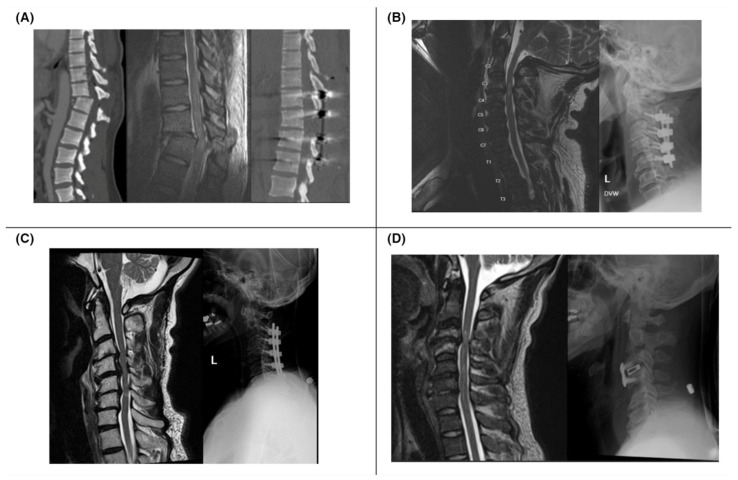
(A) Patient with lower extremity motor and sensory loss after MVC (ASIA A); CT (left) and sagittal T2 MRI (middle) show T11–12 fracture dislocation; postoperative CT shows improved alignment (right). (B) Patient with intact sensation but no motor function after fall (ASIA B); preoperative MRI (left) shows C3–4 stenoses and cord contusion; postoperative decompression and fusion from C2–5 X-ray (right). (C) Patient with 2/5 hand weakness and intact sensation (ASIA C) after fall; MRI with spinal cord contusion at C4–6 (left); postoperative X-ray with instrumented decompression and fusion (right). (D) Patient with hand paresthesia and intact motor function after fall (ASIA D); MRI with traumatic disc herniation at C3–4 (left); postoperative C3–4 anterior cervical discectomy and fusion X-ray (right).
